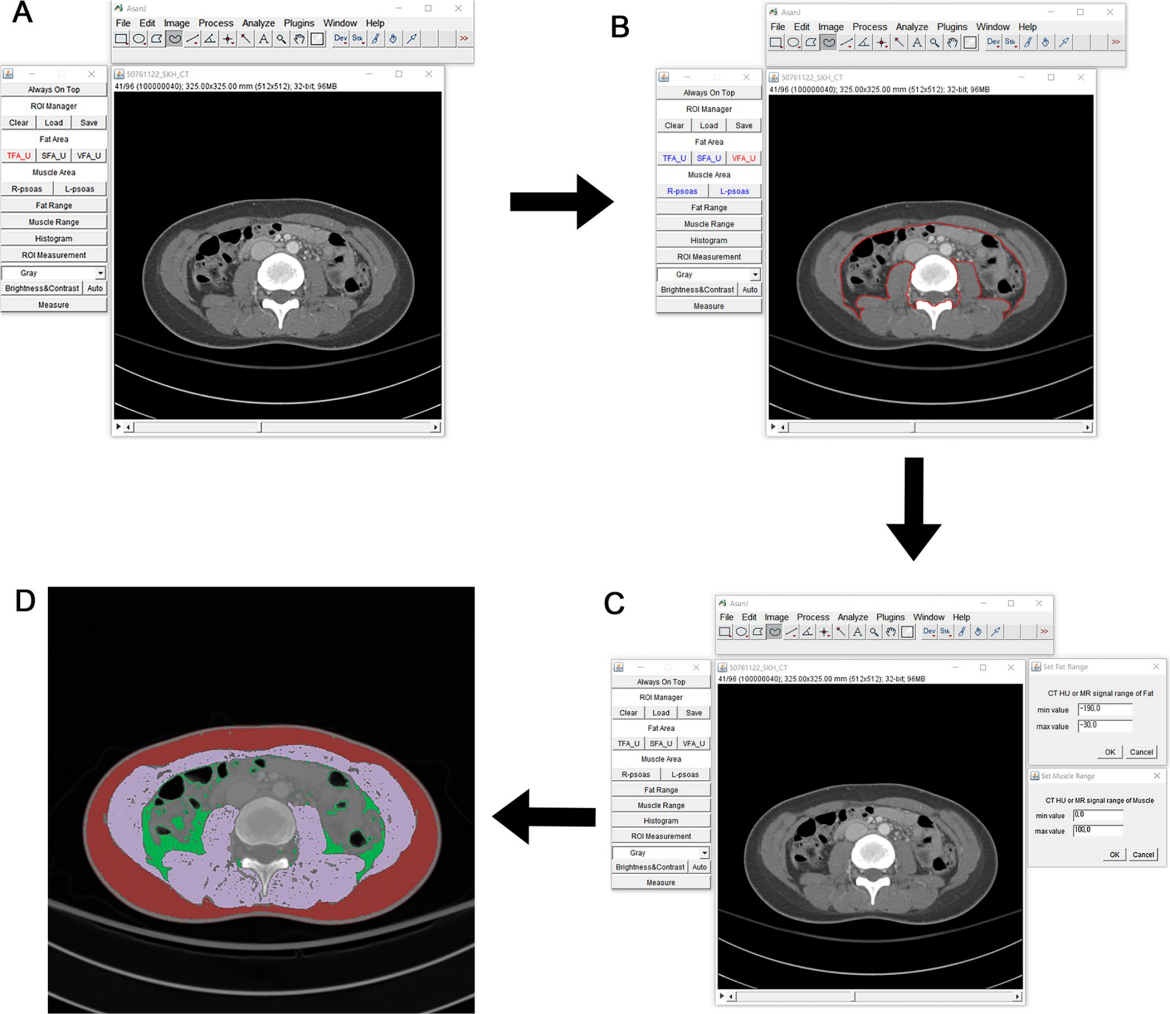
Semi-automatic segmentation for body morphometric analysis. (A) ImageJ after running the program and uploading a CT or an MR image. (B) Adjustment of the threshold of total abdominal muscle area (TAMA), total psoas muscle area (TPA), and visceral fat area (VFA). (C) Measurement of the semi-automatic regions of interest (ROI). (D) Segmentation and color mapping.
